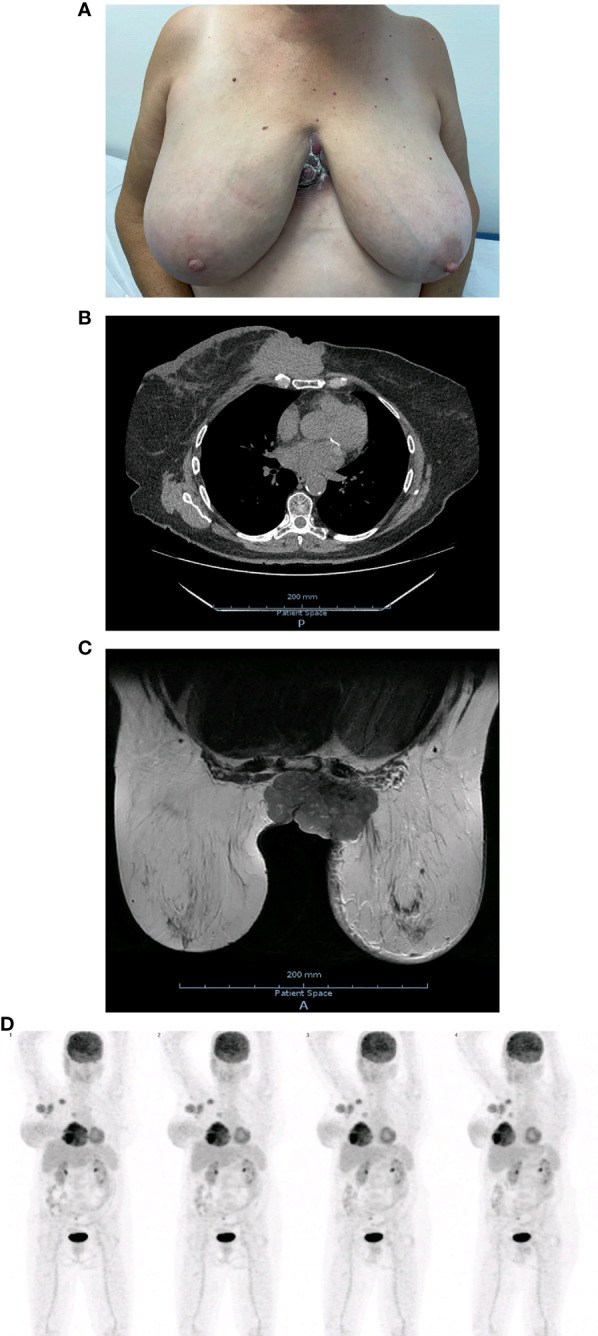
(A) Initial clinical presentation. (B) Initial chest CT. (C) Initial breast MRI. (D) Whole body on PET/CT with 18F-FDG at initial staging.
